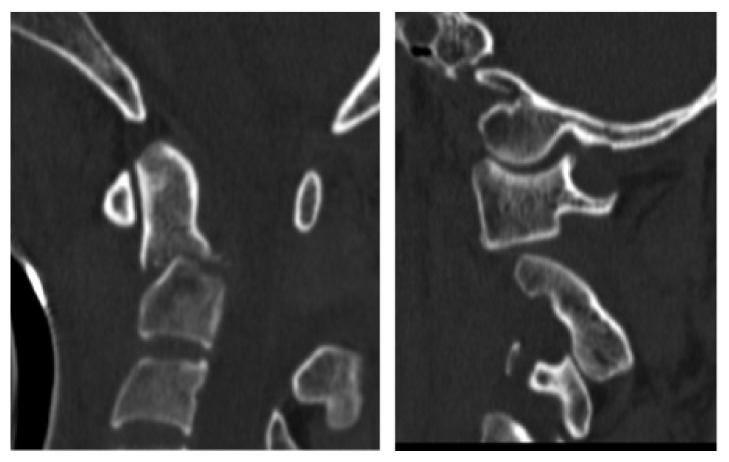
Sagittal CT reconstruction of C0 to C3, showing a low-grade displaced fracture of the odontoid process, classified as type II according to Anderson/ D'Alonzo, combined to a dissociation of C1 and C2.
